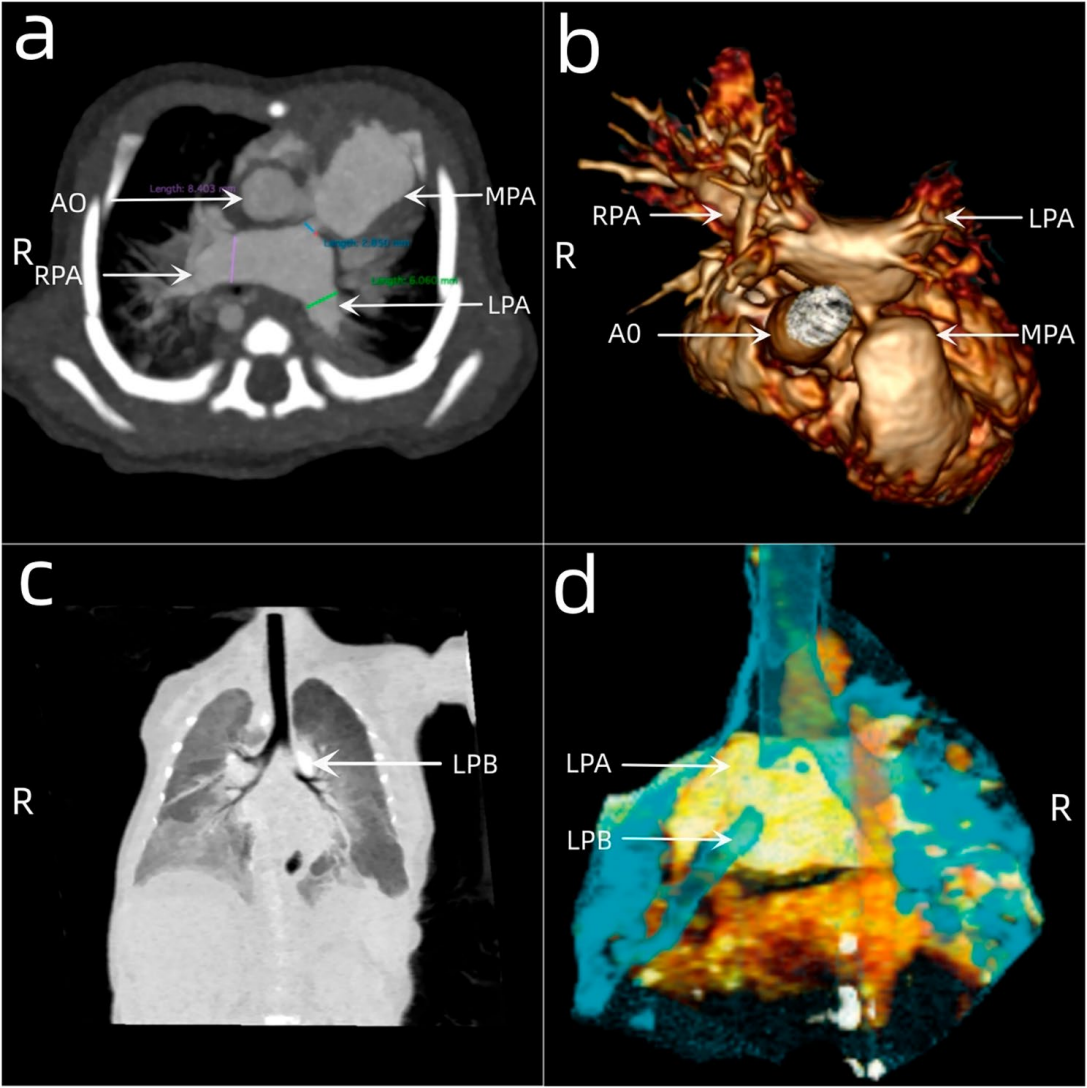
Preoperative enhanced computed tomography of the heart shows dilation in the proximal and stenosis in the distal segments of the main pulmonary artery, extreme aneurysmal expansion of the left and right pulmonary arteries, and occlusion of the left main bronchus. AO, aorta; MPA, main pulmonary artery; RPA, right pulmonary artery; LPA, left pulmonary artery; LPB, left principal bronchus
